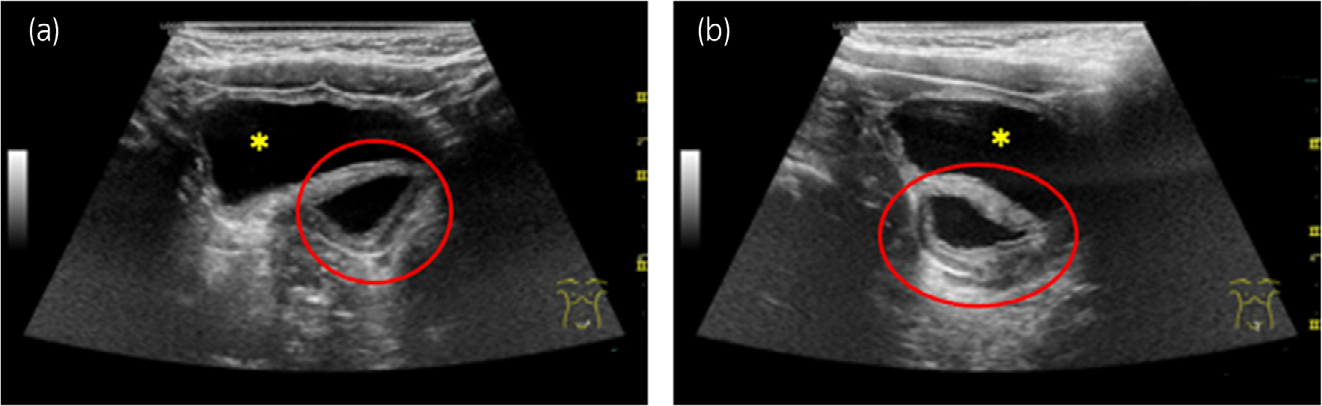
Abdominal ultrasonography. (a) Axial section; (b) sagittal section. Abdominal ultrasonography shows a 10‐mm hypoechoic lesion (red circles) on the left dorsal aspect of the bladder. The bladder is indicated by an asterisk.
